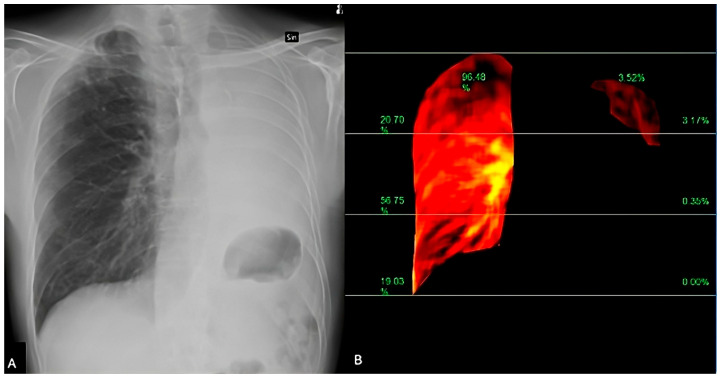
DDR perfusion assessment of a lung cancer patient, presenting dyspnea and raised D-dimer values. The traditional X-Ray (A) shows a pleural effusion extending to the apex. The perfusion study (B) demonstrated a regular perfusion of the right lung parenchyma and left apex.
